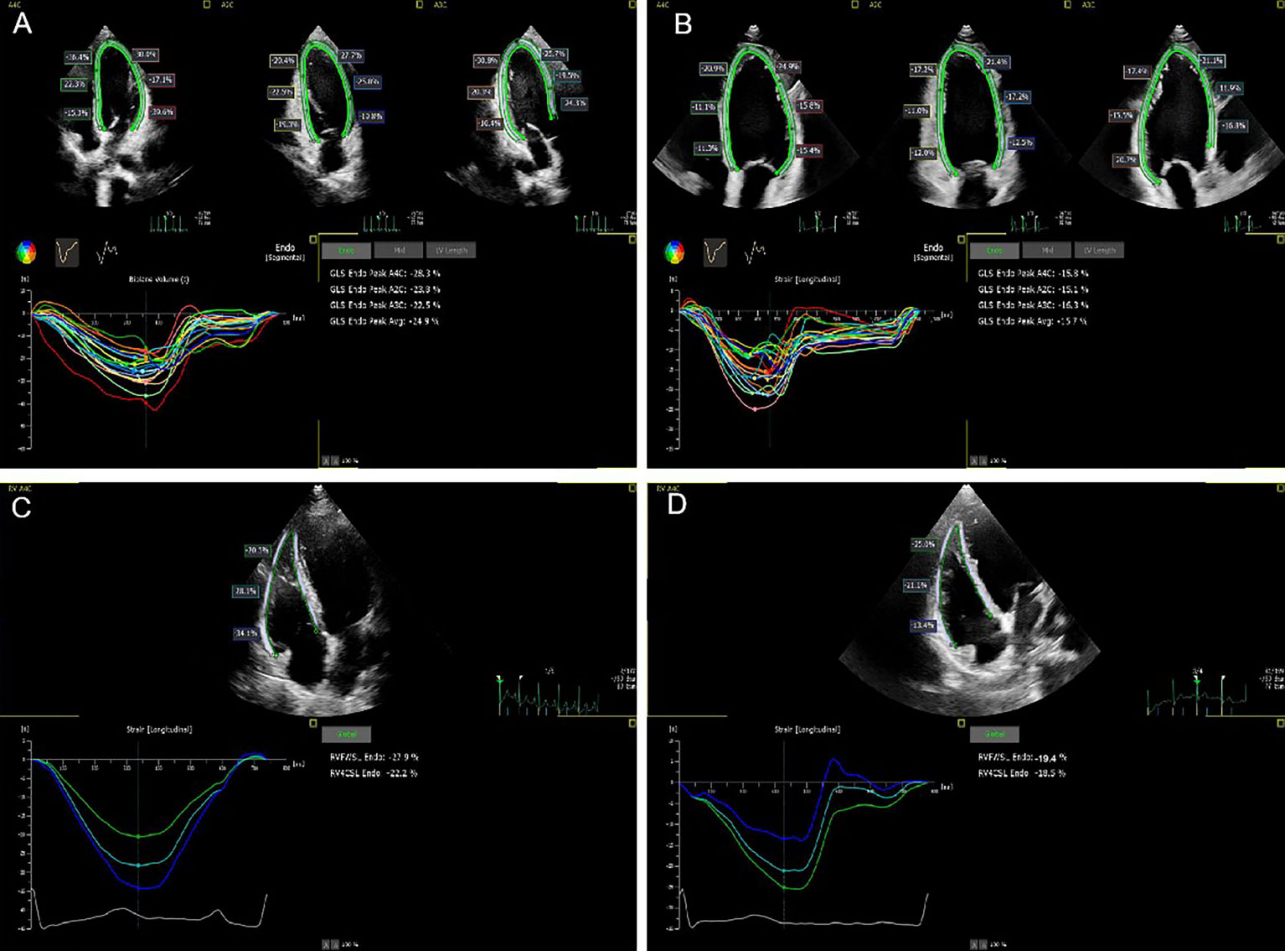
Two-dimensional strain echocardiography measurement of LVGLS and RVFWLS in obstructive sleep apnea patients. A, Upper image showing multiple cross-sectional views of the left ventricle, with green lines marking the endocardial contours. LVGLS=-24.9% and RVFWLS=-27.9%, indicating normal left and right ventricular systolic function. B, Ultrasound images showing cross-sectional views of the left ventricle, with LVGLS=-15.7% and RVFWLS=-19.4%, indicating impaired left and right ventricular systolic function. C, Cross-sectional view of the right ventricular free wall showing LVGLS=-24.9% and RVFWLS=-27.9%, indicating normal left and right ventricular systolic function. D, Cross-sectional view of the right ventricular free wall showing LVGLS=-15.7% and RVFWLS=-19.4%, indicating reduced function in both ventricles. LVGLS: left ventricular global longitudinal strain; RVFWLS: right ventricular free wall longitudinal strain.
